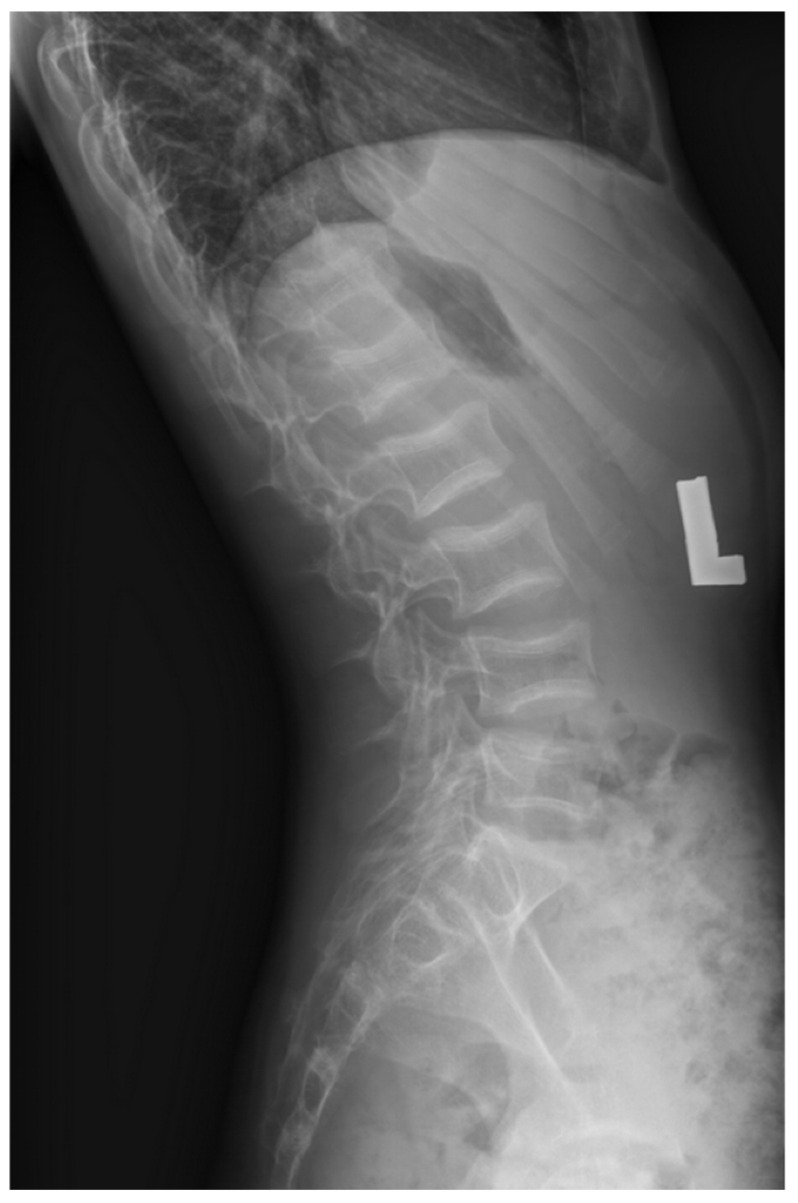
X-ray image of Patient 11 showing fractures in the vertebrae Th11-L5.
